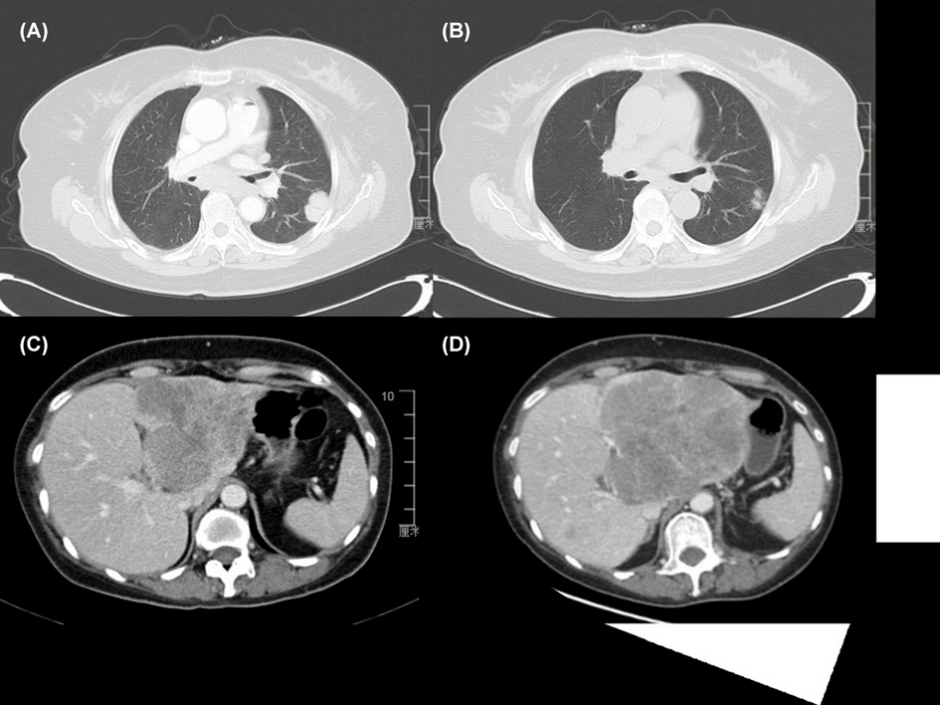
computed tomography images of typical cases The target lesion in lung at baseline was 29.1 mm (A) and decreased to 17.6 mm after six cycles in patient with nasopharyngeal cancer (B). The target lesion in liver at baseline was 98.5 mm (C) and progressed to 125.2 mm after two cycles in patient with rectal cancer (D).
